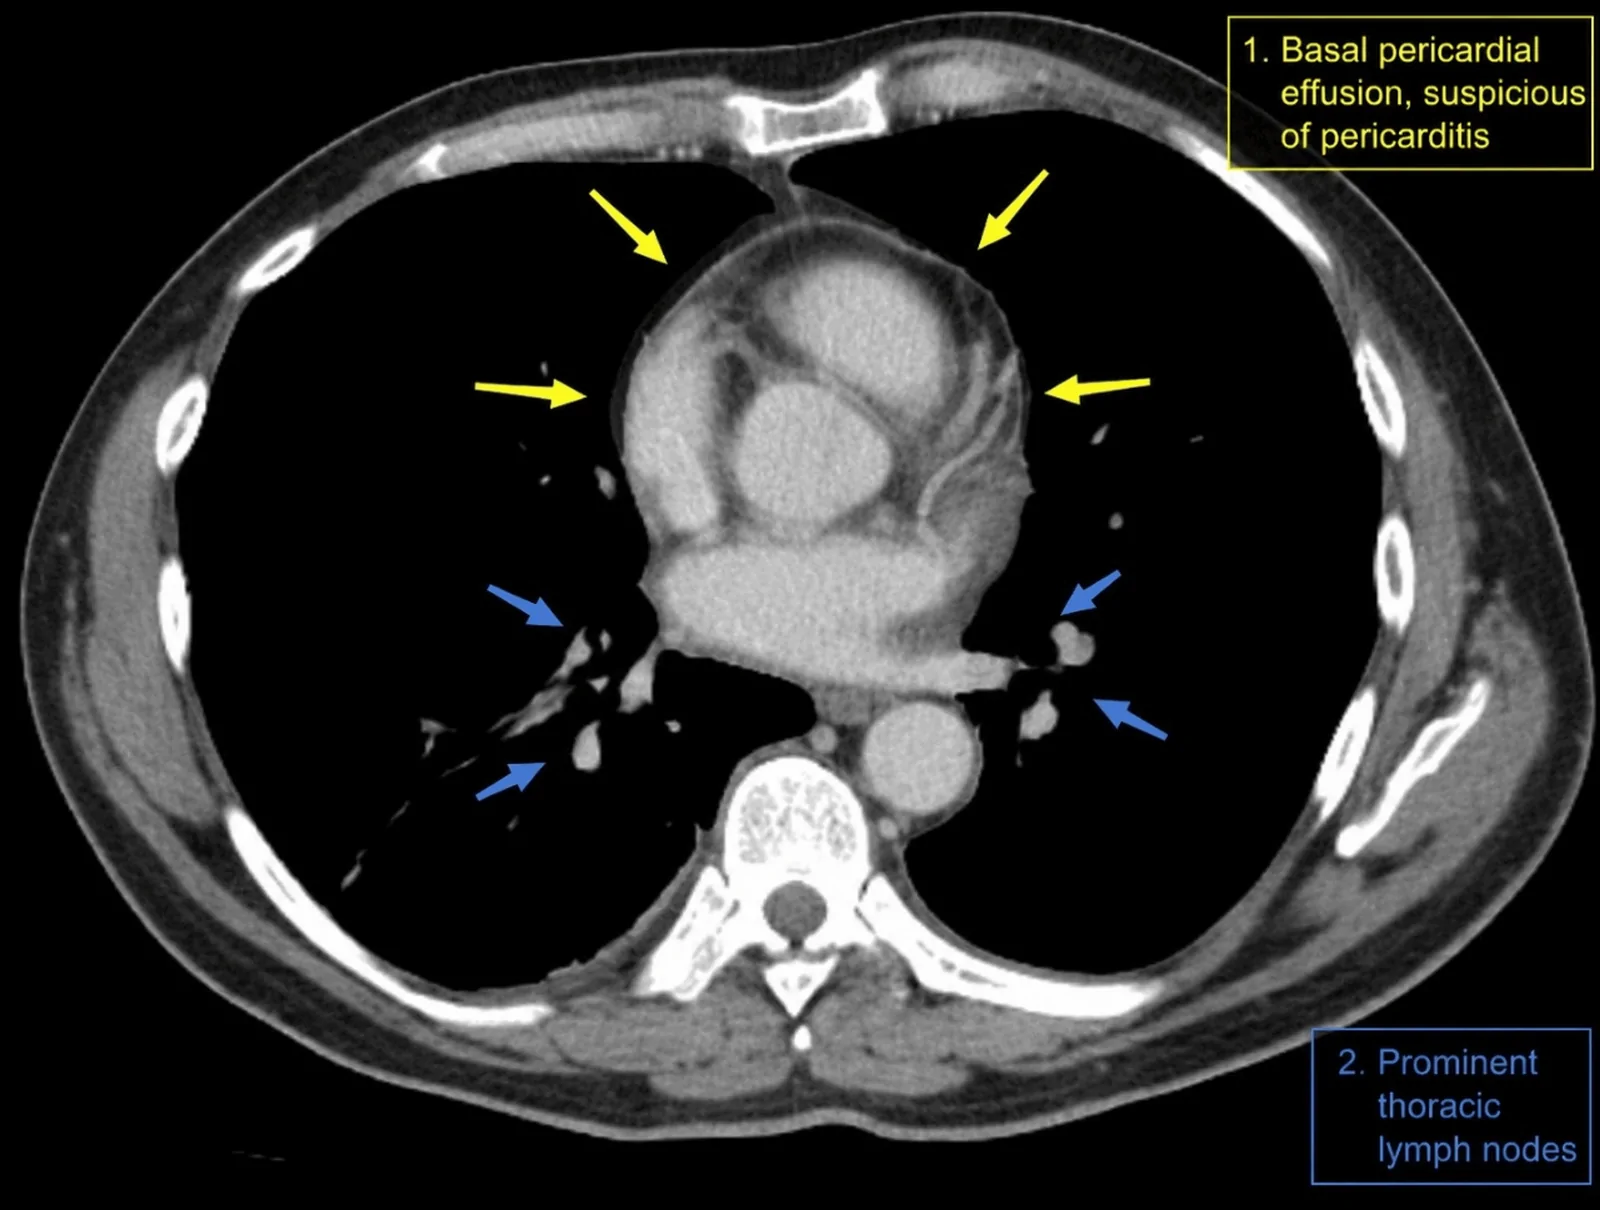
CT of the chest, abdomen and pelvis demonstrating pericardial effusion up to 1.3 cm with pericardial enhancement and thoracic lymphadenopathy CT: computed tomography
